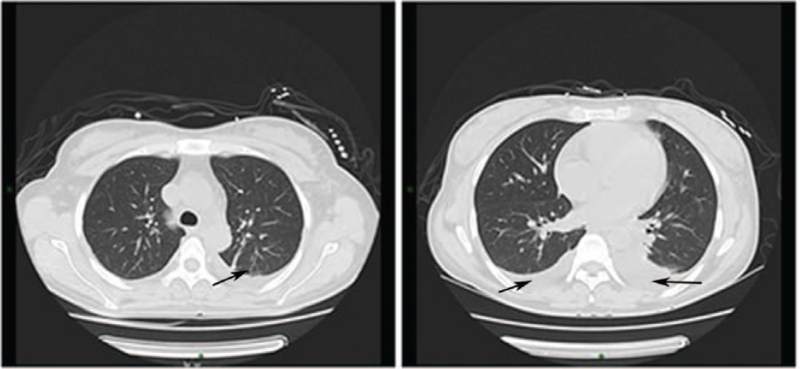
Chest CT on Day 8. Bilateral pleural effusion and local atelectasis; ground glass density shadow and strip shadow in adjacent lung field were considered to be associated with chronic inflammation.
